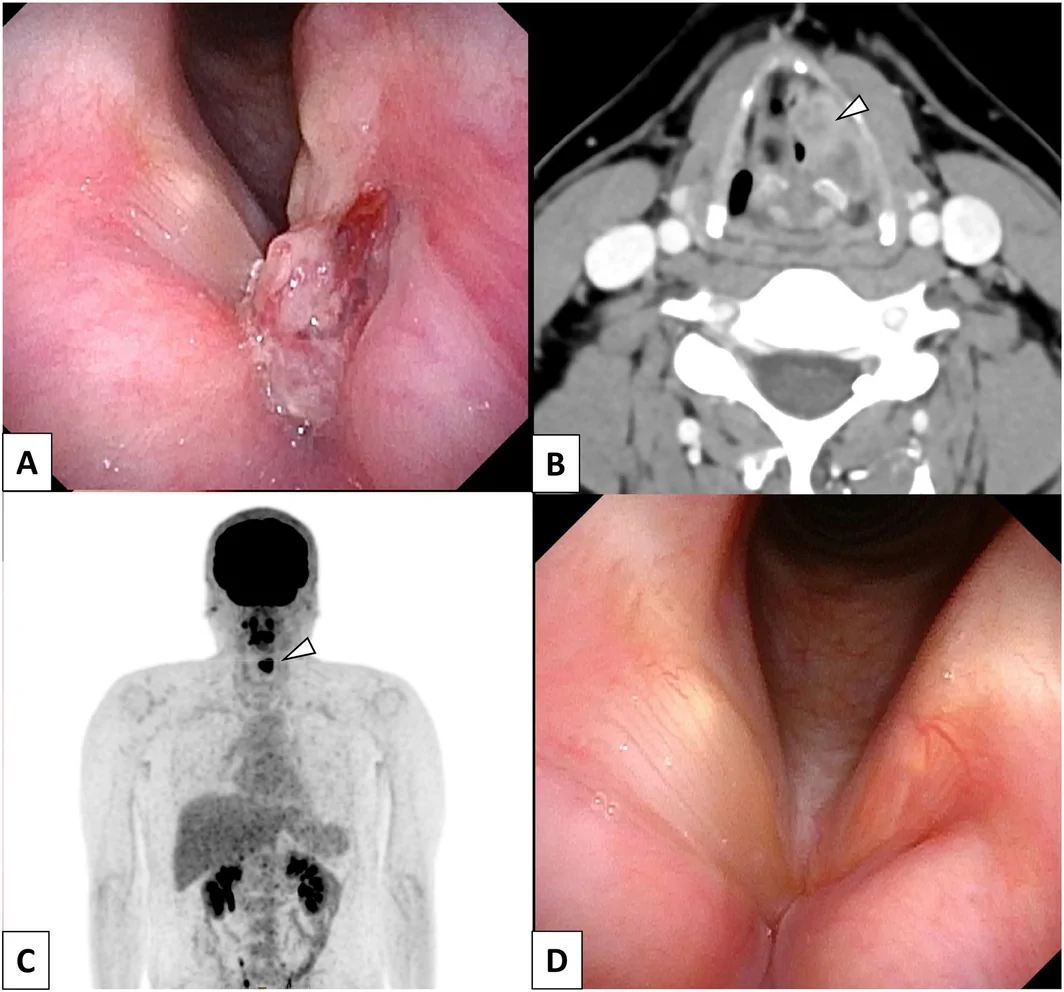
Endoscopic and imaging findings at initial presentation and after definitive therapy. (A) Flexible laryngoscopy demonstrating a tumor extending from the anterior commissure to the left true vocal fold. (B) Contrast‐enhanced CT showing a mass involving the left true vocal fold (white arrow) without evidence of thyroid cartilage invasion. (C) PET/CT demonstrating increased fluorodeoxyglucose (FDG) uptake confined to the primary laryngeal lesion (white arrow), with no evidence of nodal or distant metastasis. (D) Flexible laryngoscopy after completion of definitive chemoradiotherapy showing complete resolution of the primary lesion. CT, computed tomography; PET, positron emission tomography.
